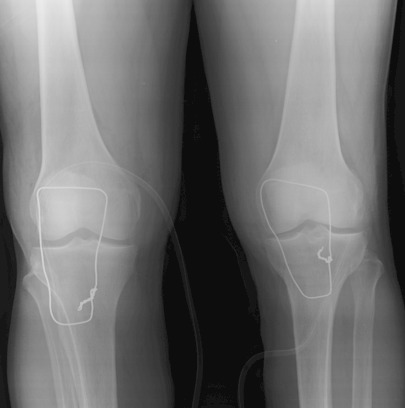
Radiographie de face après réparation du tendon rotulien et protection par cadrage, les deux rotules sont au même niveau
